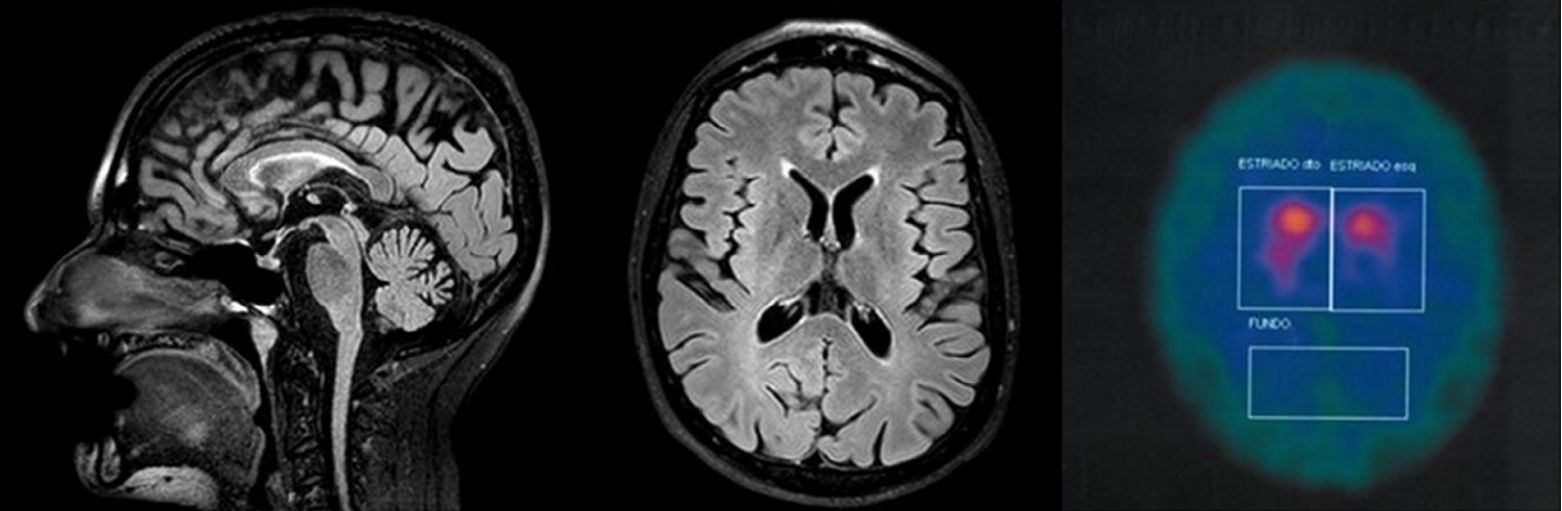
Magnetic resonance imaging and dopamine transporter scan of case 2. Sagittal (A) and transversal (B) fluid attenuated inversion recovery magnetic resonance imaging sequences show generalized brain atrophy with prominent parietal and cerebellar involvement as well as an “ear of the lynx sign” on the left (B). Dopamine transporter single‐photon emission computed tomography demonstrating reduced tracer uptake predominantly on the left.
